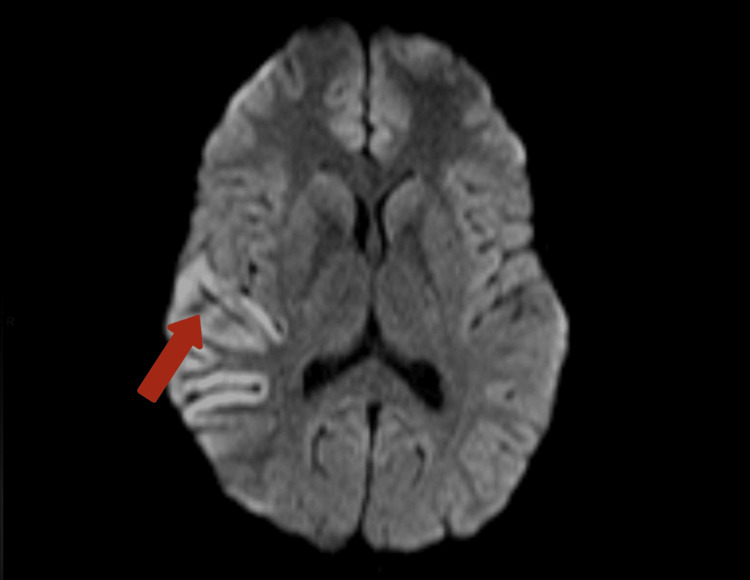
MRI image-DWI showing fairly defined gyriform areas of DWI hyperintensities with corresponding subtle ADC hypointensities. This is an MRI-T2 weighted image indicating an area of hyperintensity in the cortical region of the right-temporo parietal lobe. DWI: diffusion-weighted imaging; ADC: apparent diffusion coefficient
